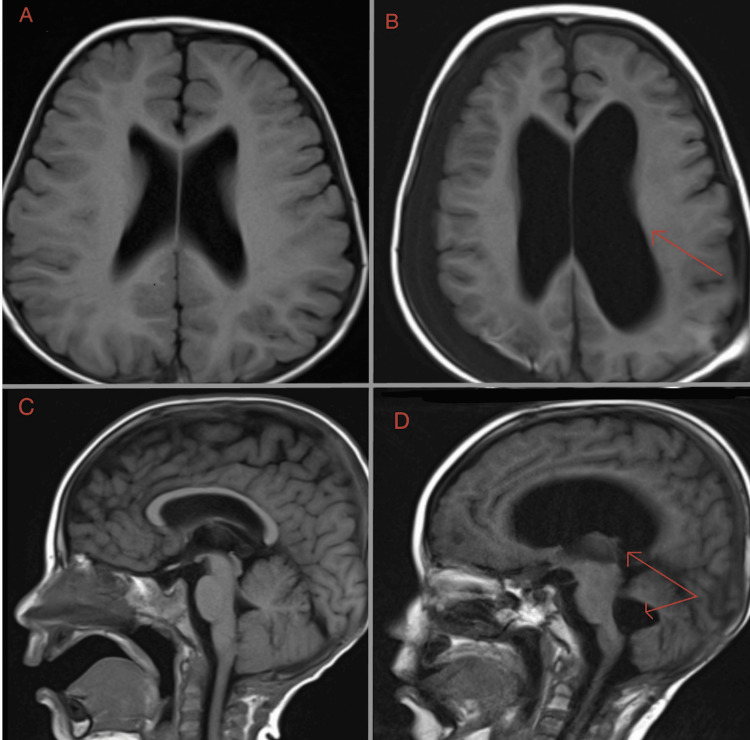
Serial neuroimaging demonstrating progressive ventriculomegaly in a child with suspected shunt malfunction (A) Baseline axial MRI following tumor resection demonstrating normal ventricular caliber. (B) Axial MRI during admission demonstrating marked dilation of the lateral and third ventricles consistent with acute ventriculomegaly (red arrow). (C) Baseline sagittal MRI demonstrating normal ventricular anatomy and third ventricular size. (D) Sagittal MRI obtained during clinical deterioration demonstrating enlargement of the ventricular system, particularly the third ventricle (red arrow). MRI: Magnetic resonance imaging.
